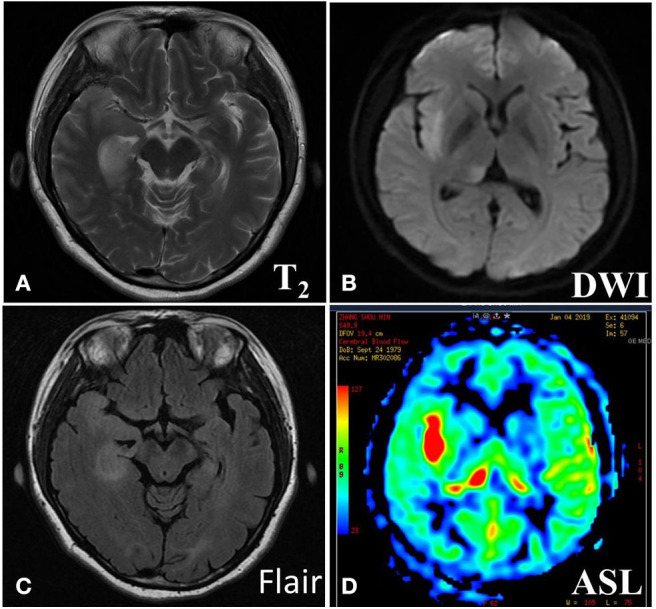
Brain MRI (A) showed abnormal signal in right temporal and insular lobe, thalamus. On T2WI (B) and T2Flair (C) sequences, the right temporal and insular lobe, right thalamus showed slightly higher abnormal signal, the local cortex was slightly swollen, and on the DWI sequences, slightly higher signal was seen. On T2Flair sequence (C), there was high abnormal signal in the right hippocampus and no obvious abnormal signal in the left hippocampus. Arterial spin labeling (ASL) sequence (D) showed significant hyperperfusion in the right temporal and insular lobe, thalamus.
